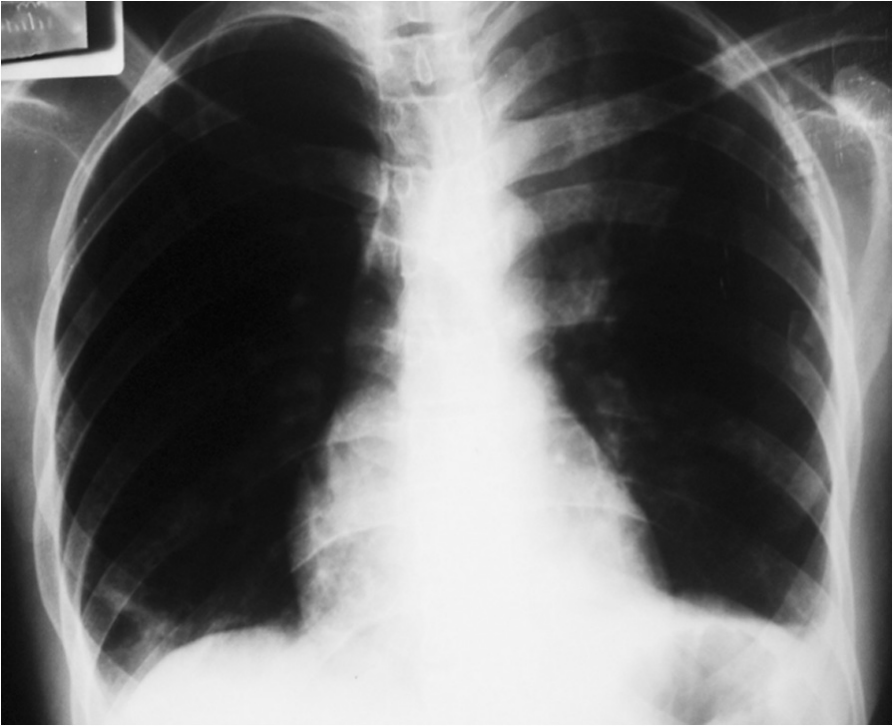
Chest X-ray showing left lung re-expansion.
